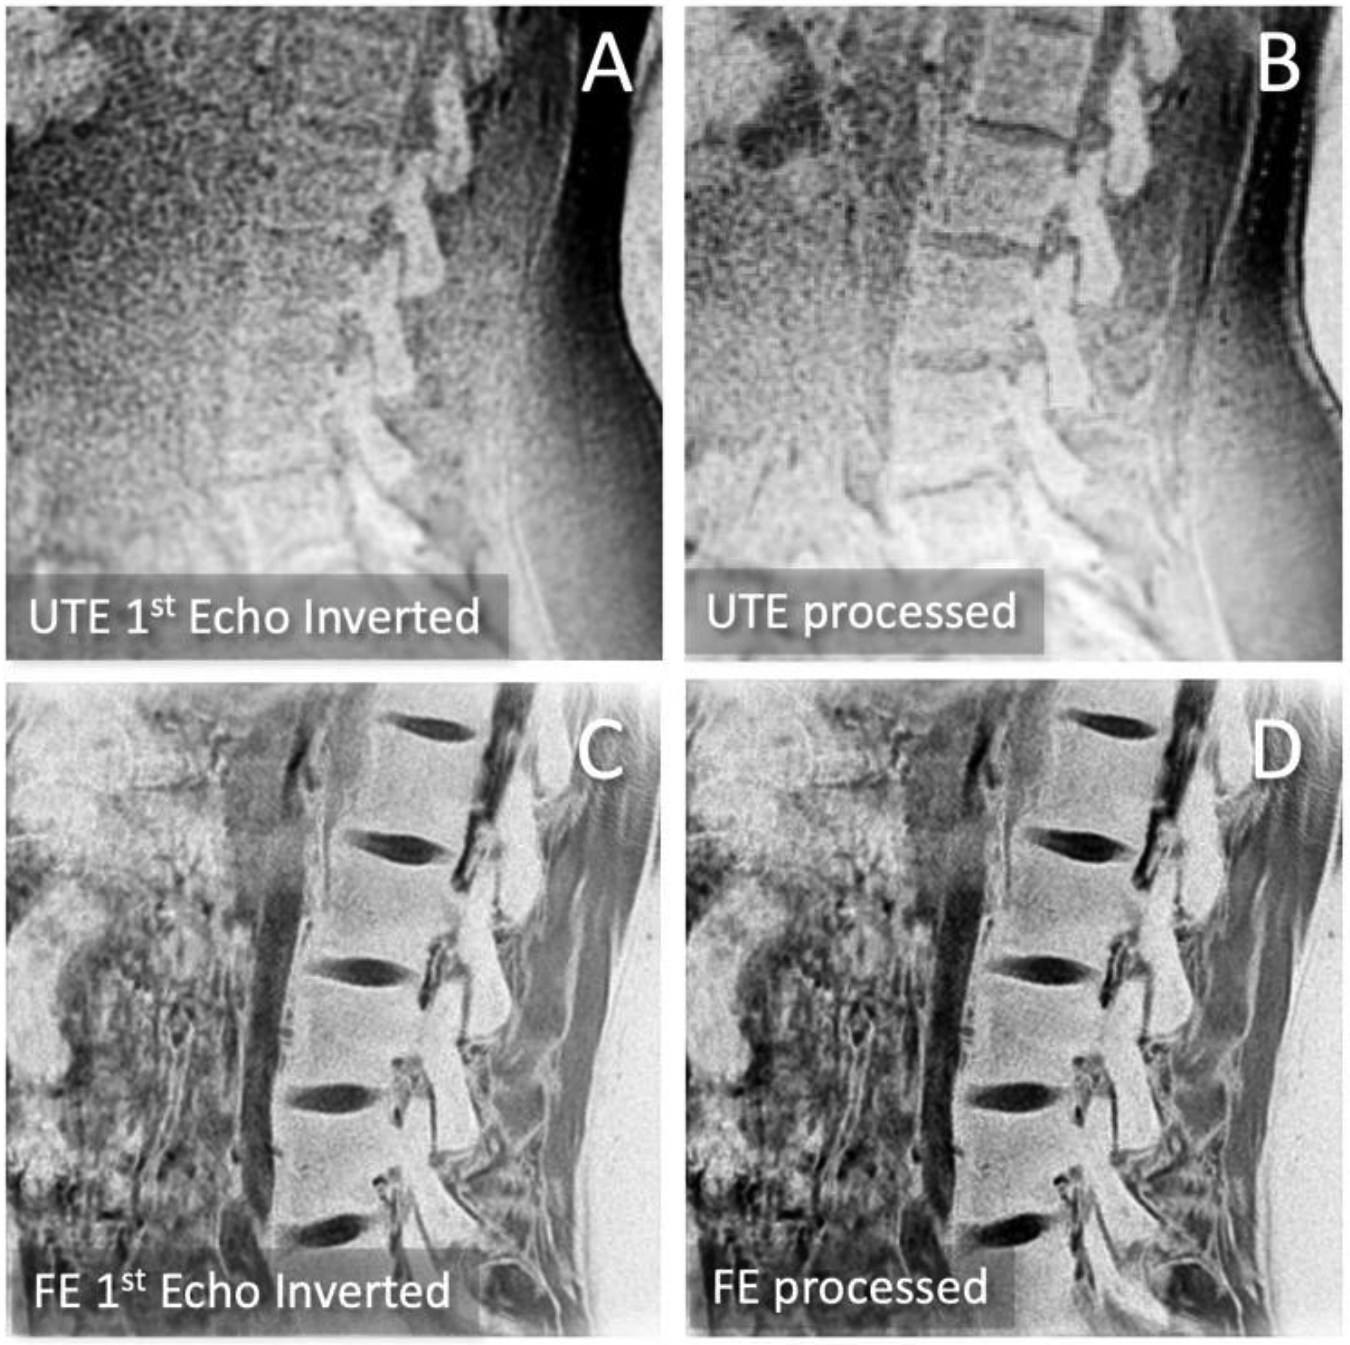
Processed images to create CT-like contrast from (A) UTE 1^st^ echo image, (B) UTE multiecho images, and (C) FE 1^st^ echo image, and (D) FE multiecho images. Differences between sequences (UTE vs. FE) and improvements in contrast and image quality from multiecho processing are apparent.
